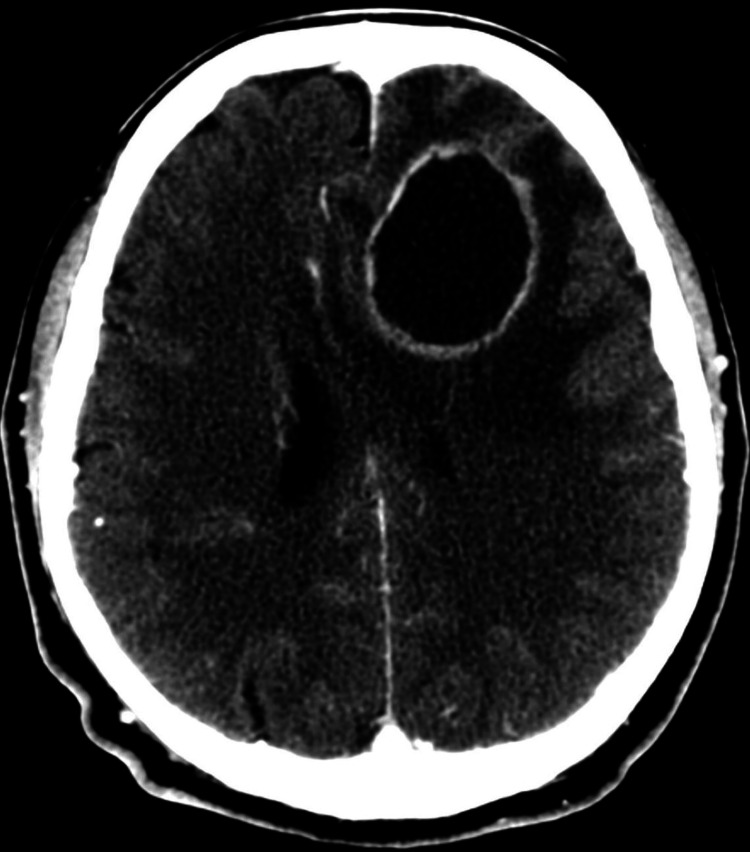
Tuberculoma on head CT scan. Enhanced head CT scan in axial views of an HIV patient with a left frontal tuberculoma showing hypodense necrotic central area with ring enhancement, and perilesional severe edema with subfalcine herniation (Courtesy of Doctor Felipe Alva-Lopez, co-author).
